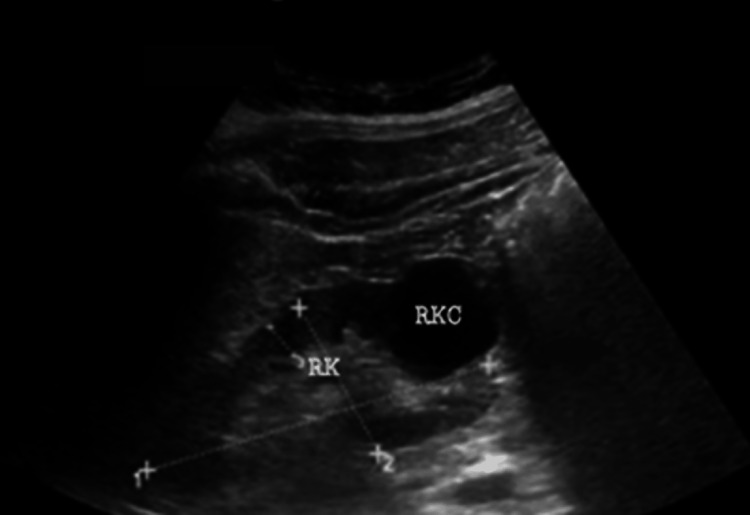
Abdominal ultrasound. RK = right kidney; RKC = right kidney cyst
